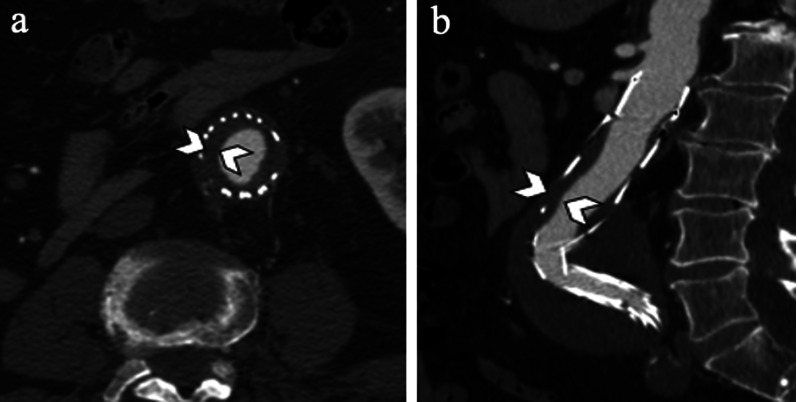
81-year-old man with stent-graft thrombosis. a Axial and b sagittal CT angiography images show non-enhancing concentric thrombus (arrowheads) inside the lumen of the endograft
